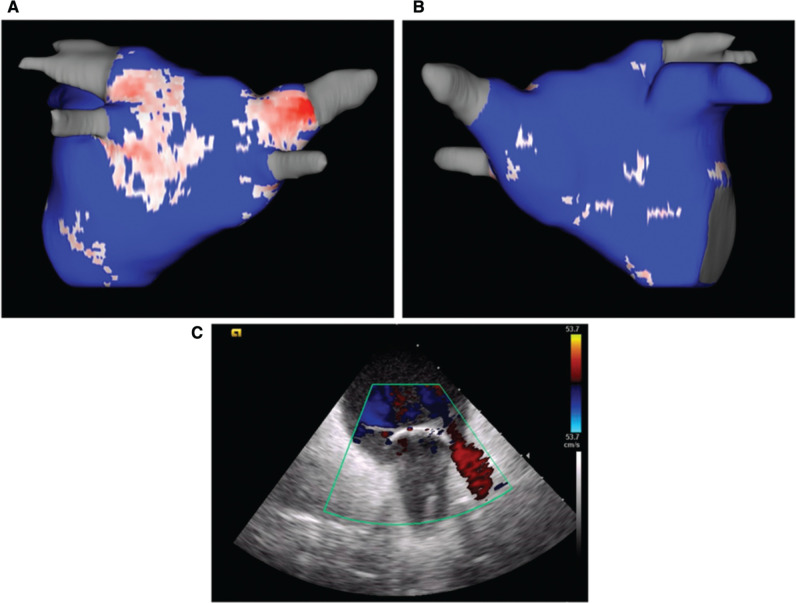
Postablation left atrial fibrosis map showing a postablative scar in red on the posterior (A) and anterior (B) left atrial walls with gaps on the anterior wall corresponding to an area of incomplete seal of the cryoablation balloon seen on intracardiac echocardiography images (C). Subsequent repeat ablation showed leak anteriorly with successful ablation in this area for pulmonary vein reisolation.
